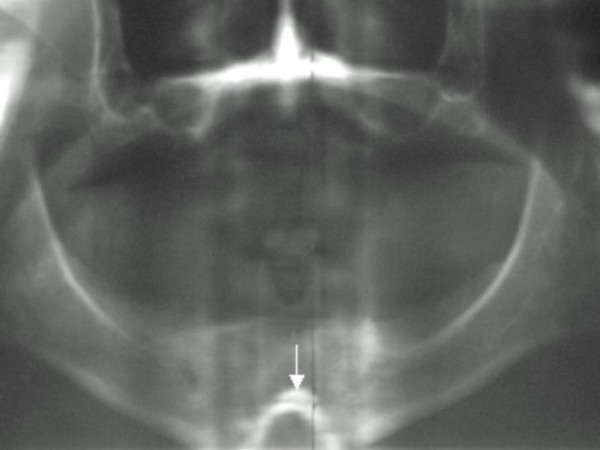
Radiographic pantogram showed total anodontia, in addition there was inverted V shaped dysplastic mental protuberance (arrow).
